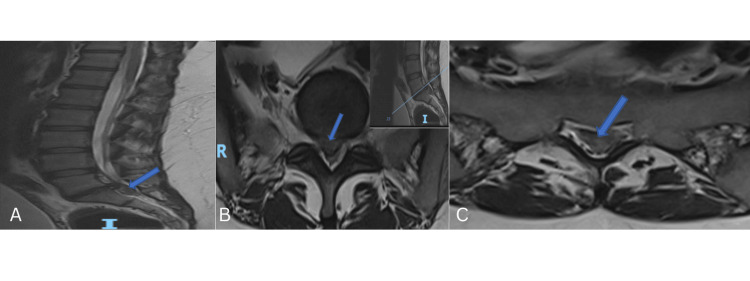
MRI of the lumbar spine. (A) Sagittal T2 sequence with blue arrow showing an isointense L5-S1 disc herniation with caudal migration. (B, C) Consecutive axial T2 sequences with blue arrows showing an isointense central disc extrusion filling the dural sac.
